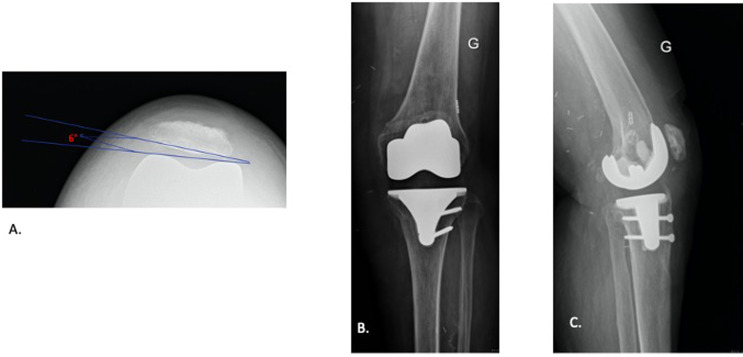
Post-MPFL reconstruction. Post-operative X-rays of the patient from Figure 1 were taken at 12 weeks post-surgery. (A) Sky-line view demonstrating patella now centered with a patella tilt of 6°. (B) AP X-ray, the endobutton can be seen sitting flush on the lateral cortex. (C) Lateral profile demonstrating the tibial-tubercle osteotomy and tunnel position. The osteotomy is not yet fully united.
